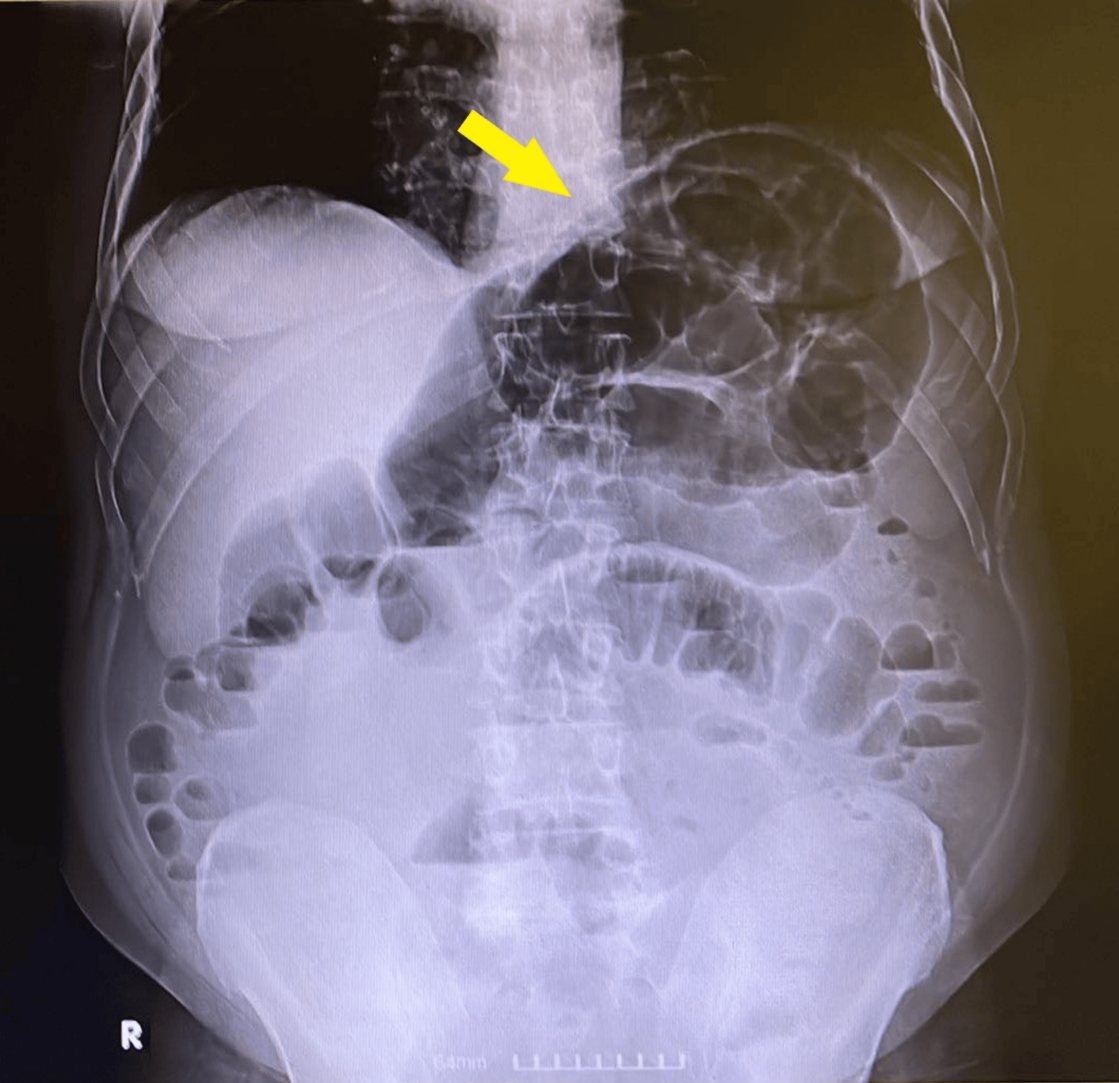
Abdominal X-ray showing signs of diffuse dilation of the small intestine, without a clear transition point (yellow arrow).
